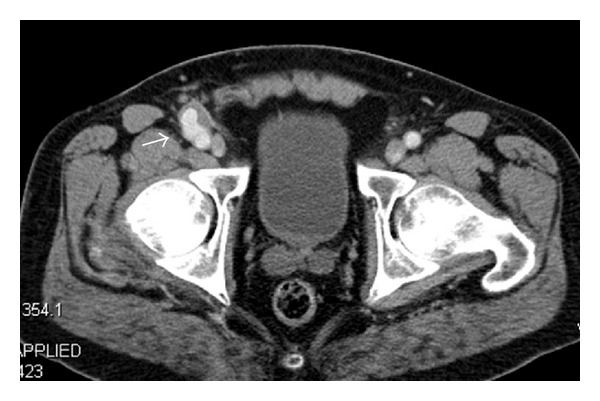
Larger aneurysma spurium of the right common femoral artery (arrow) with a wide neck which was detected by CT imaging three months after percutaneous EVAR, requiring surgical cutdown.
